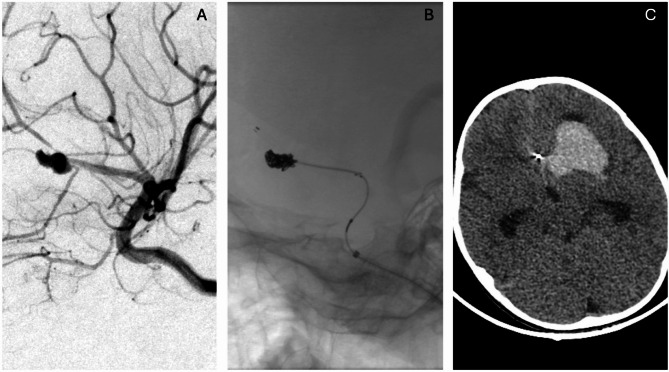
A: A roadmap image shows the preoperative subtracted image, A1 artery size is decreased possibly vasospasm after subarachnoid hemorrhage B: A single stent-assisted coiling is completed in native images. C: Postoperative CT demonstrates no increase in hemorrhage volume and no signs of acute hydrocephalus
